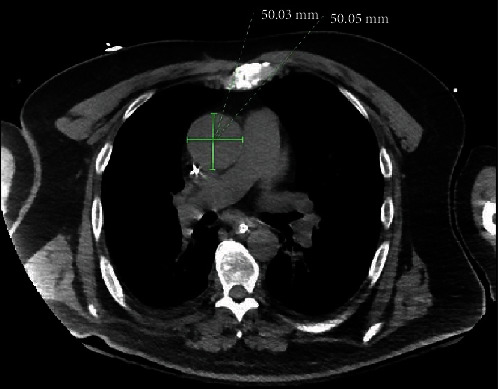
Soft-tissue axial view of CT chest demonstrating dilated aortic root.
